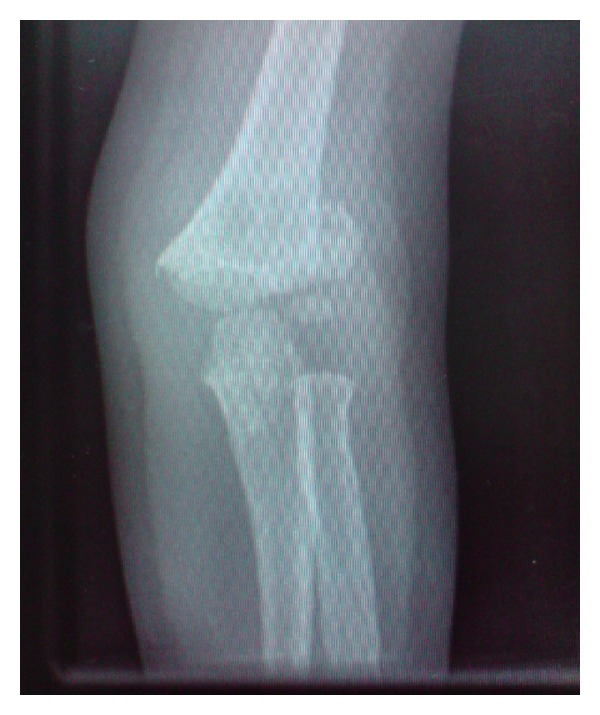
Preoperative AP radiograph.
